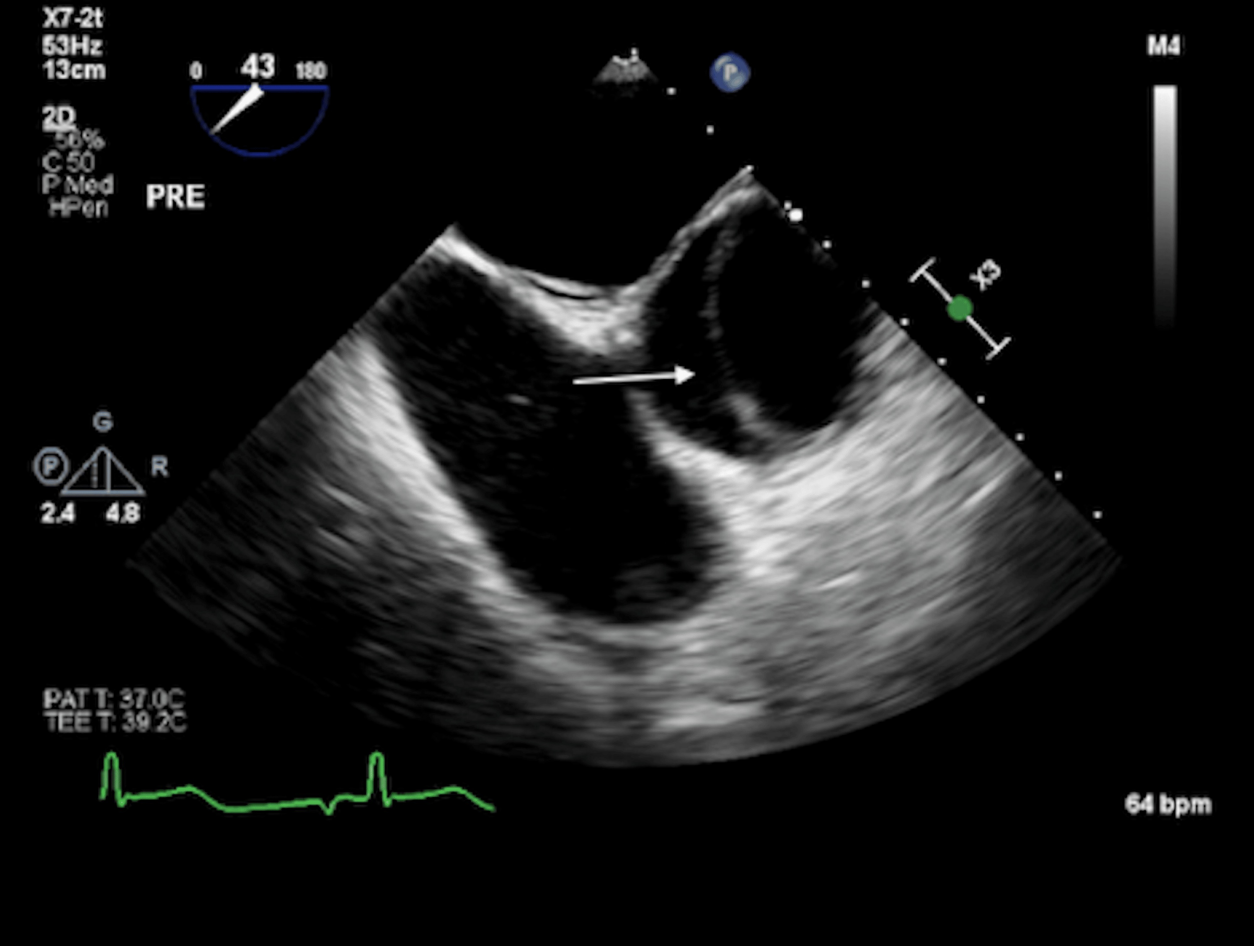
Intraoperative transesophageal echocardiography showing type A aortic dissection with dissection flap as highlighted by the white arrow
